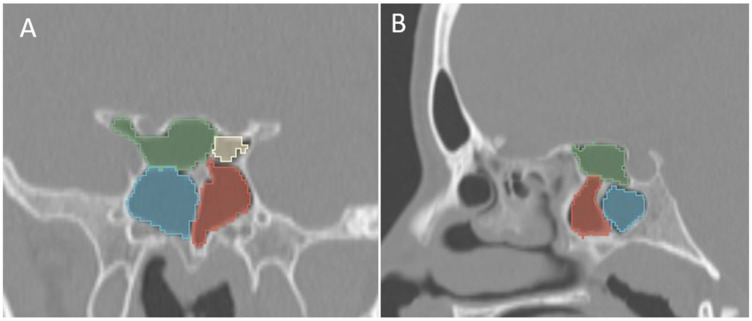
Coronal (A) and sagittal (B) reformatted CT images of a 13-year-old boy with bilateral Onodi cells (green and yellow) above the sphenoid sinuses (blue and red).
